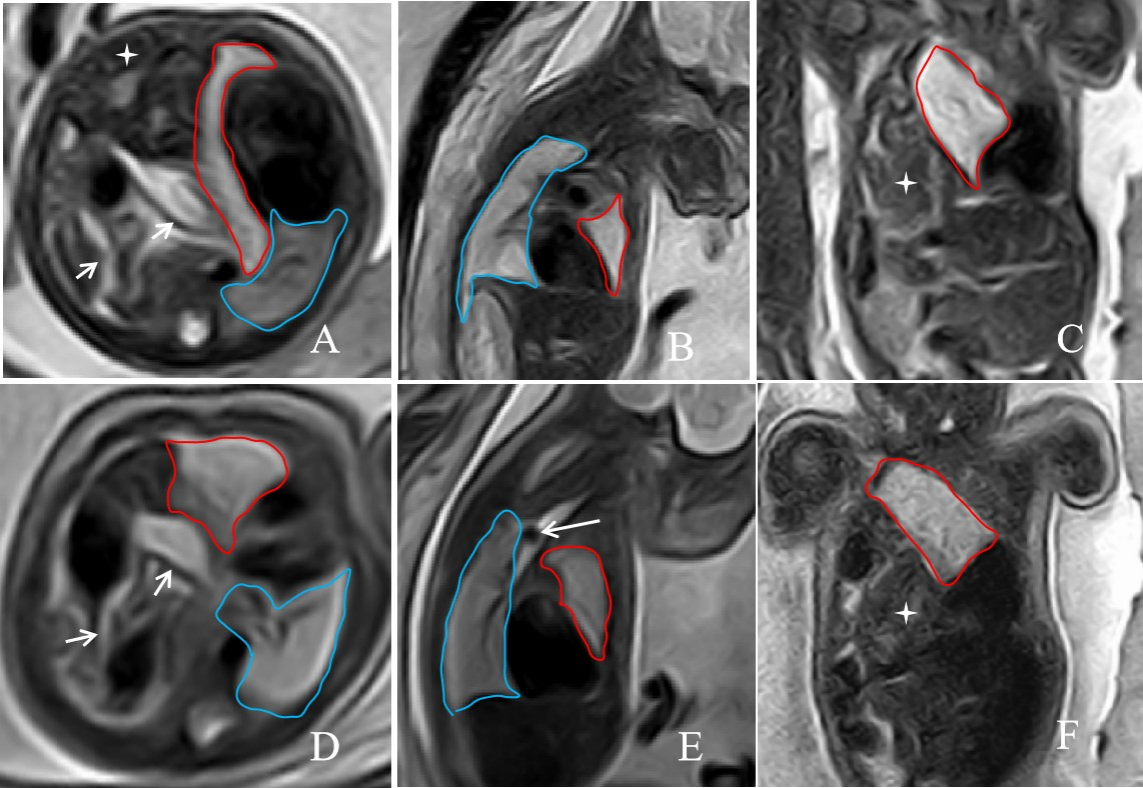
A 28-years-old pregnant woman, the fetus with left CHD combined with congenital pulmonary cystadenoma on prenatal MRI. The axial, sagittal, and coronal T2-weighted images at 28w1d of gestation(A, B and C) before FETO treatment, and at 35w of gestation after FETO treatment(D, E and F) showed abdominal organs, such as the stomach, intestines, and partial left lobe of liver, herniated into the chest cavity (short arrow and cross Star). A sagittal T2-weighted image (E) showed that a low-signal balloon was inserted into the trachea above the carina during FETO surgery(E, long arrow). The area delineated by the red line represents the congenital cystadenoma occurring in the left lung, while the area outlined by the blue line represents the normal right lung.
